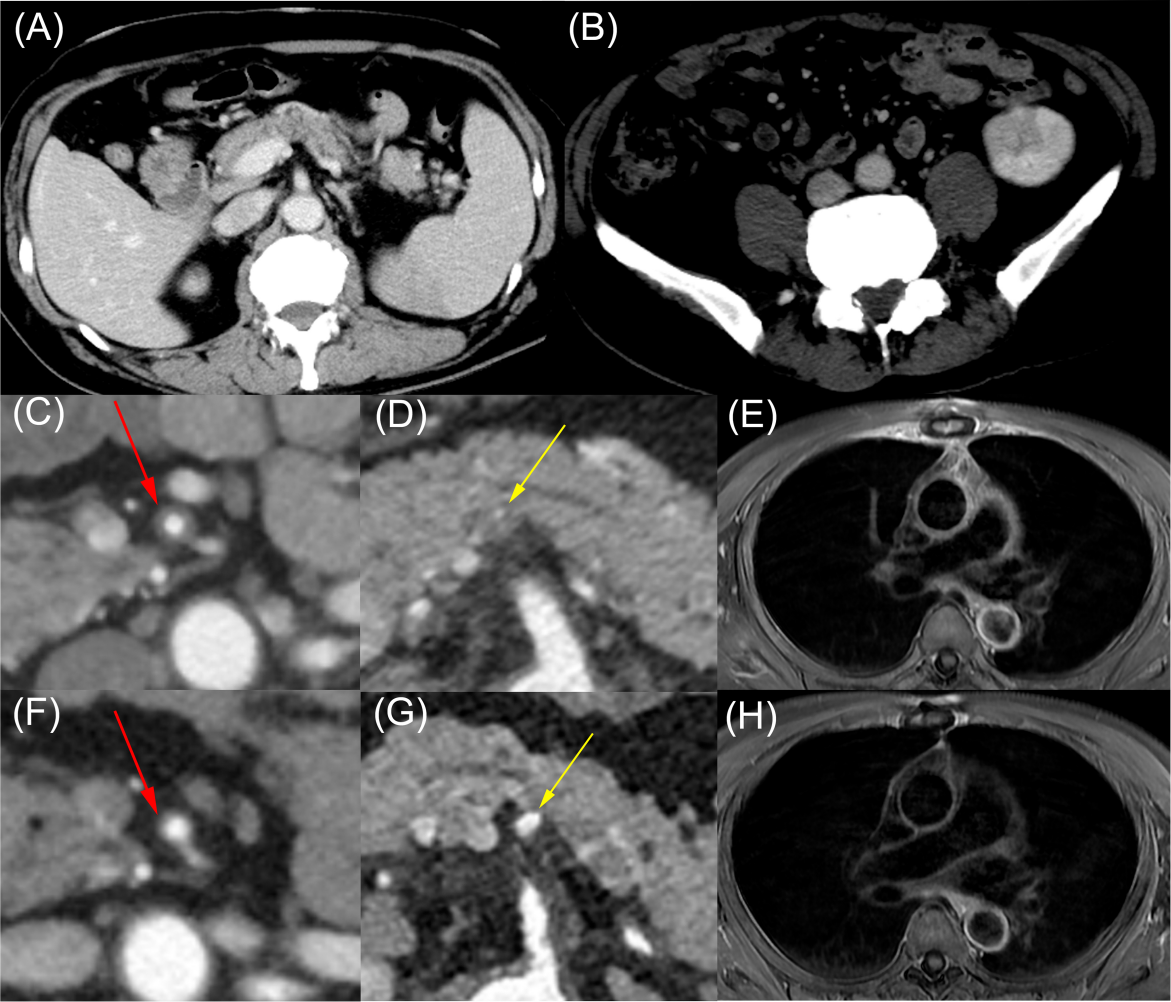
Imaging findings of *JAK2* V617F-positive essential thrombocythemia with multivessel vasculitis before and after ruxolitinib therapy. Contrast-enhanced computed tomography (CT) and magnetic resonance imaging (MRI) revealed visceral infarctions and systemic vasculitis before treatment, with marked improvement after ruxolitinib therapy. (A, B) Contrast-enhanced CT showing splenic infarction (A) and partial left renal infarction (B). (C–E) Pretreatment contrast-enhanced CT and MRI demonstrating vasculitic changes in medium- and large-sized vessels, including wall thickening with stenosis of the superior mesenteric artery [(C), red arrows], wall thickening with stenosis at the origin of the splenic artery [(D), yellow arrows], and vascular wall thickening with contrast enhancement of the ascending and descending aorta (E). (F–H) Follow-up contrast-enhanced CT and MRI obtained 6 months after ruxolitinib therapy demonstrated marked improvement in vasculitic changes in the superior mesenteric artery [(F), red arrows], the origin of the splenic artery [(G), yellow arrows], and the aorta (H).
